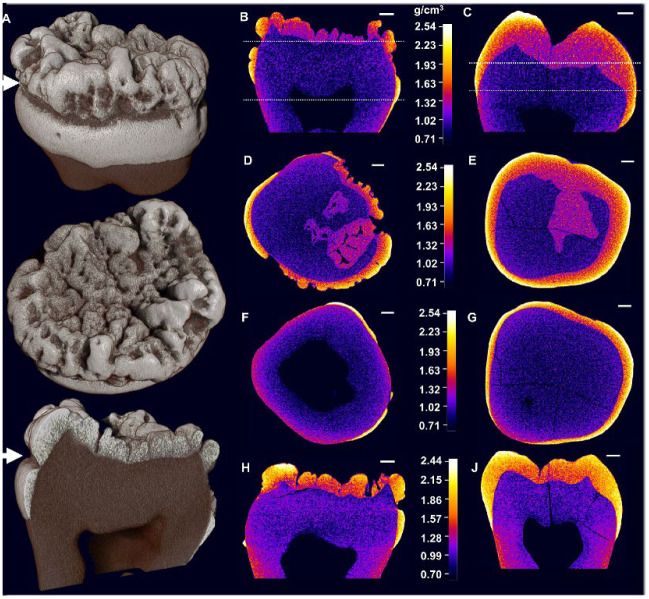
High-resolution x-ray computed tomography (CT) scan calibrated false-color plots. (A) Three-dimensional CT reconstruction of laminin subunit beta 3 (LAMB3) tooth 1 shows the multitude of cusps and the groove encircling the crown and separating the occlusal and cervical enamel (white arrows). (B, C, H, J) Longitudinal views through each tooth. Dotted lines on panels B and C indicate the positions of the transverse views through the coronal and cervical enamel shown in panels D–G. Transverse views through the (D, E) coronal enamel and (F, G) cervical enamel. A, B, D, F: LAMB3 tooth 1. C, E, G: control tooth 1. H: LAMB3 tooth 2. J: Control tooth 2. Note that the undulating enamel-dentine border in the affected LAMB3 teeth is not atypical; it is present in other CT sections of control teeth, as shown in Appendix Figure 6. Scale bars: 1 mm.
